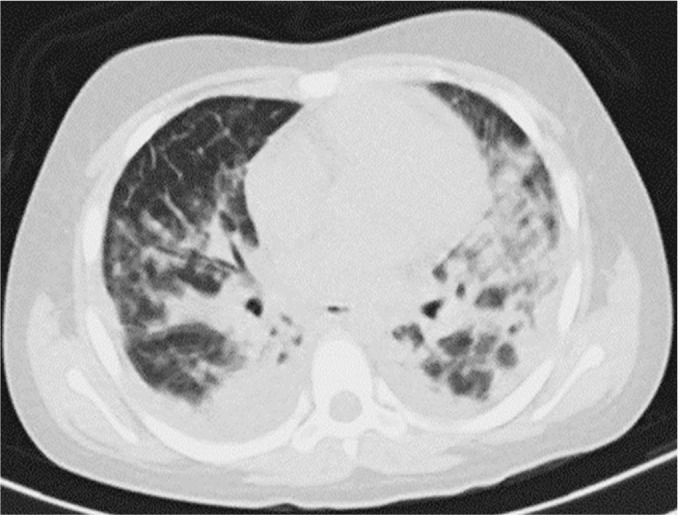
Pulmonary computed tomography performed on admission.
